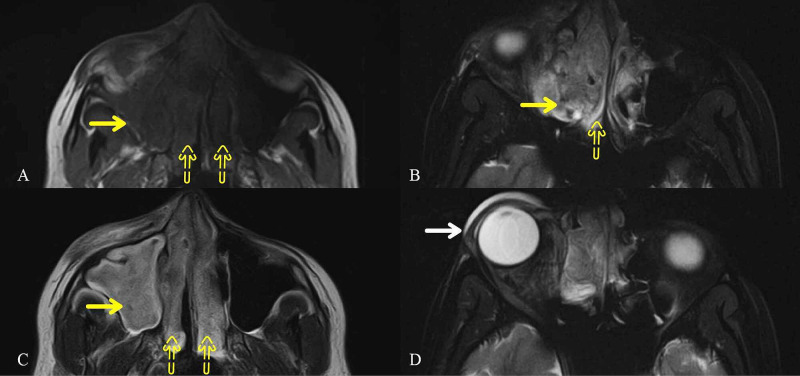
Orbital Cuts of MRI Brain almost complete opacification involving the right maxillary (solid yellow arrows) and bilateral ethmoid (yellow dotted arrows) sinuses, appearing (A) hypointense on T1 and (B, C) hyperintense on T2-weighted images. (D) There is mass effect causing slight proptosis of the right eyeball with preorbital soft tissue swelling (solid white arrow). MRI: magnetic resonance imaging
